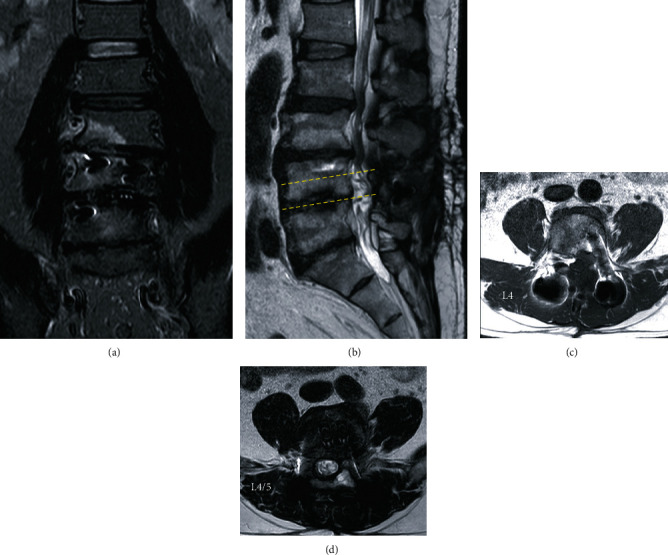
Magnetic resonance imaging on short tau inversion recovery (a), T2-weighted (b, d), and T1-weighted (c) at 1 year after surgery. The epidural lipoma was completely resected while the dural canal and nerve roots were decompressed. The endplate degeneration finding at the L4/5 level was improved.
